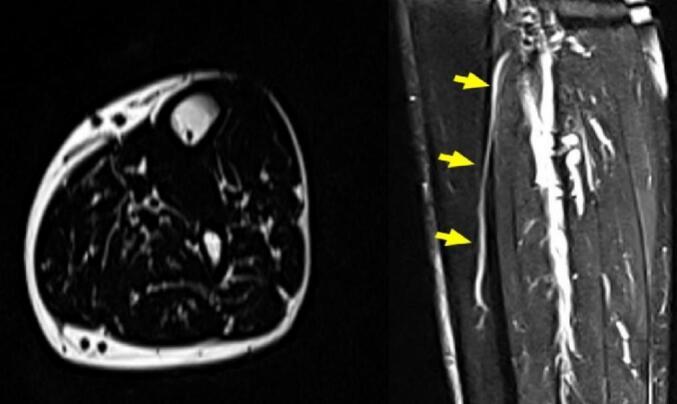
MRI; shows an intraosseous vein within the tibial medullary canal with T2 hyperintensity and posterior cortical communication. No other abnormalities, such as bone marrow edema, soft tissue masses, or stress fractures, were detected.
